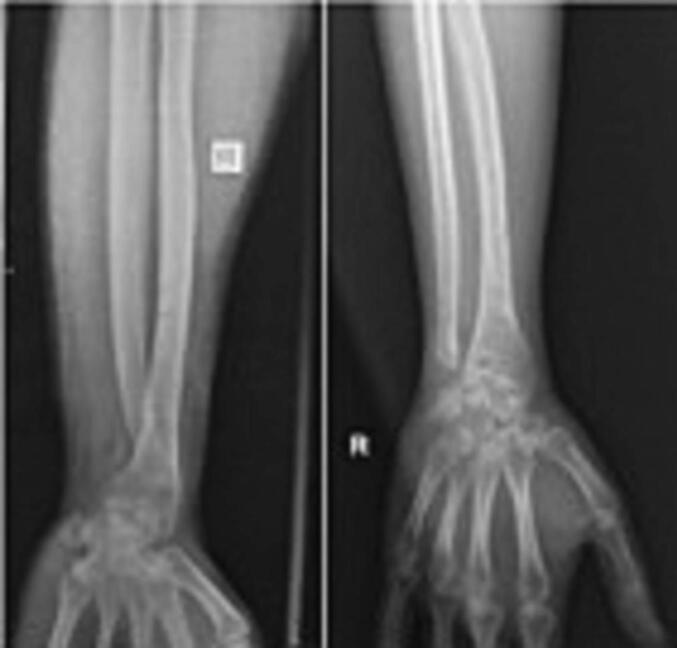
Right forearm X-ray examination before (left) and after (right) surgery shows the improvement of the DRUJ.
